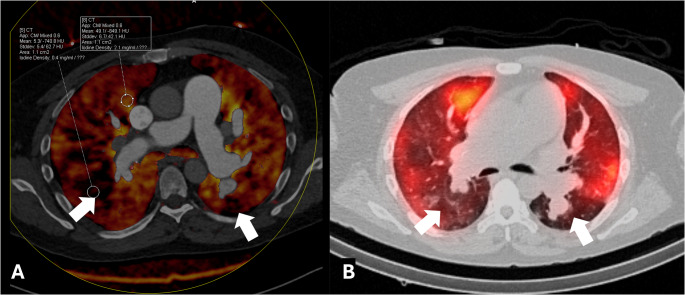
Photon-counting–detector CT iodine map vs. VQ scan. A 65-year-old man with chronic thromboembolic pulmonary hypertension. A Photon-counting–detector CT iodine map demonstrates multifocal lobar perfusion defects (white arrow). Quantitative iodine measurements show markedly reduced iodine density within the perfusion defect (0.2 mg/mL) compared with normally perfused lung (1.9 mg/mL). B Same-day V/Q SPECT perfusion imaging in the axial plane demonstrates corresponding perfusion defects in the same regions (white arrow), confirming true perfusion abnormalities
